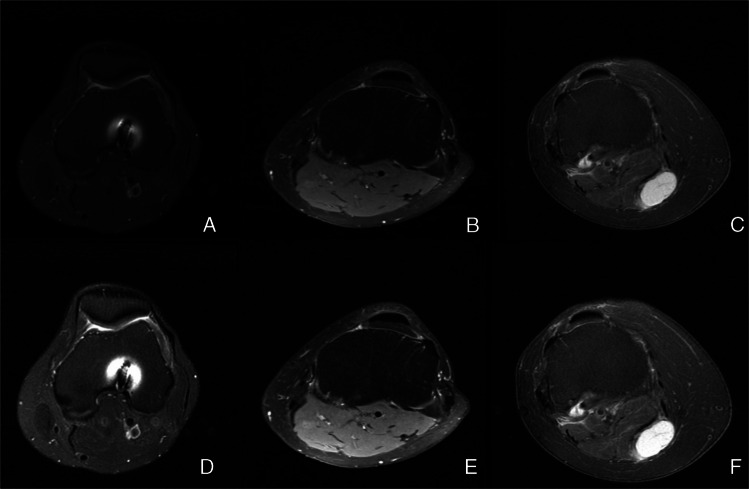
Axial MR images before and after (A/D, B/E, C/F) percentile-based intensity normalization, resulting in more consistent brightness, contrast, and saturation for downstream analysis. After image normalization, the popliteal artery (arrowhead) in figures A/D demonstrates increased conspicuity. Figures A and D demonstrate susceptibility artifacts (straight arrow) related to prior surgical hardware, while figures C and F show a Baker’s cyst (curved arrow). These cases were intentionally included to ensure dataset heterogeneity and to better reflect the variability encountered in knee MRI examinations from the general population
